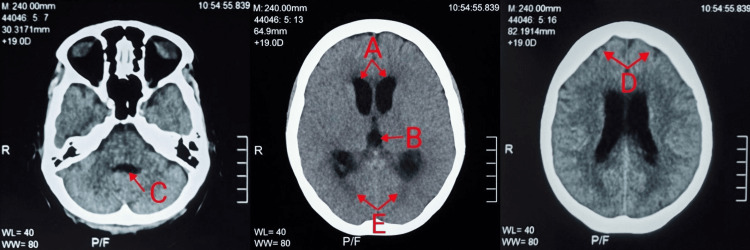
CT brain imaging revealed dilated bilateral lateral ventricles (A) and third ventricles (B), alongside a prominent fourth ventricle (C). There was associated effacement of the bilateral cerebral sulci (D), suggesting communicating hydrocephalus with concurrent cerebral oedema. Periventricular hypodensities were also noted, likely representing transependymal CSF seepage (E).
